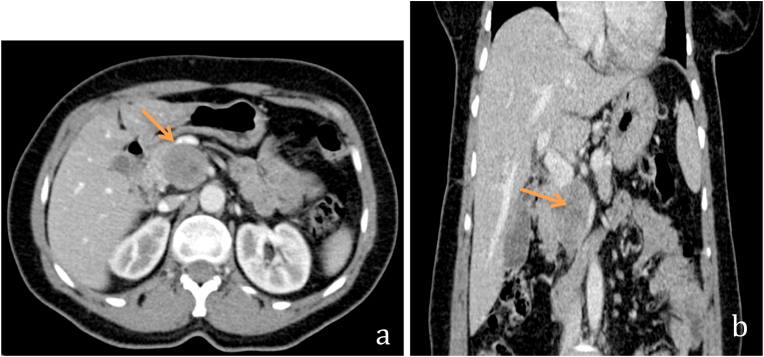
Axial (a) and coronal (b) sections of contrast-enhanced CT scan of the abdomen showing a focal mass with inhomogeneous enhancement in the pancreatic head (arrow).
